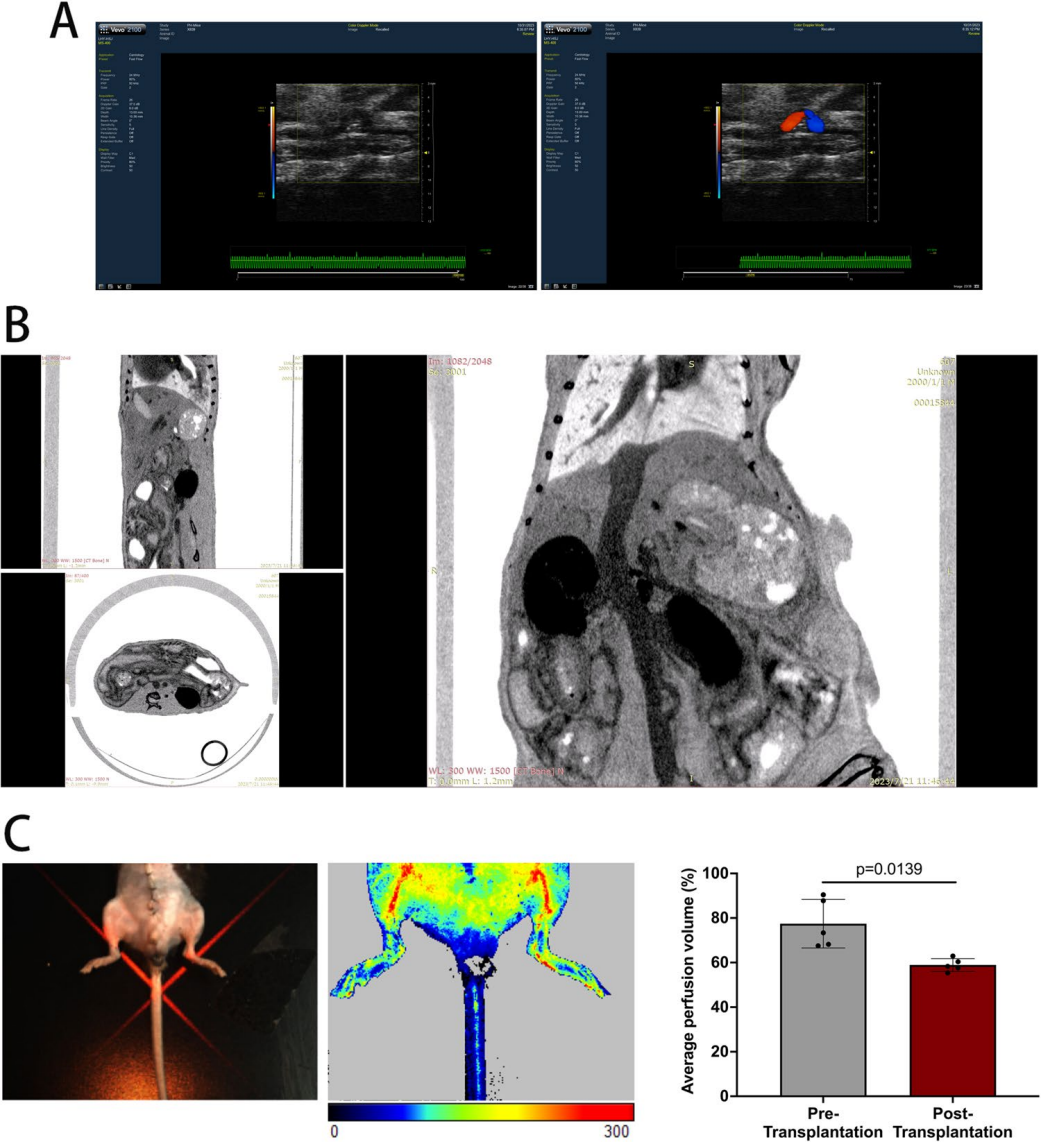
In vivo imaging of aortic arch graft for patency assessment. A Two-dimension and Doppler ultrasound imaging showing the aortic arch graft and the blood flow direction; B micro-CT imaging of coronal, sagittal, and vertical planes of the aortic arch graft; C imaging of lower limb blood flow 1 week after transplantation and its average perfusion volume compared with the pre-transplant group (*N* = 5 in each group), data were analyzed by *t* test; red arrows: abdominal aorta; blue arrows: inferior vena cava; white arrows: aortic arch graft
